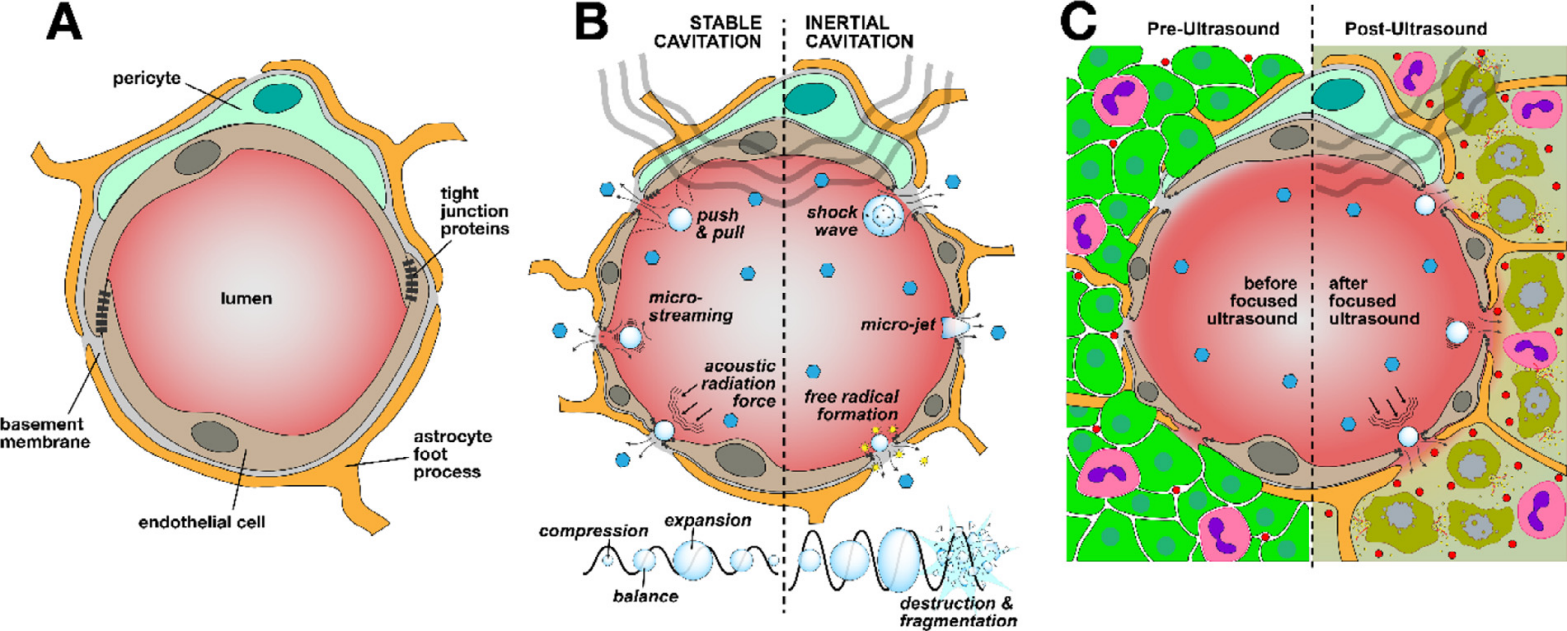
Exposure to LIFU alters BBB/BTB permeability through transient disruption of tight junction proteins. (A) Brain endothelial cells in a healthy BBB inhibit paracellular transport through presence of tight junctions formed by the neurovascular unit. (B) Harmonic and sub-harmonic oscillations of the microbubbles under ultrasonic exposures cause loosening of the tight junction proteins through stable or inertial cavitations. (C) In brain tumors LIFU causes increased delivery of chemotherapeutics to tumors causing additional tumor cell apoptosis.
